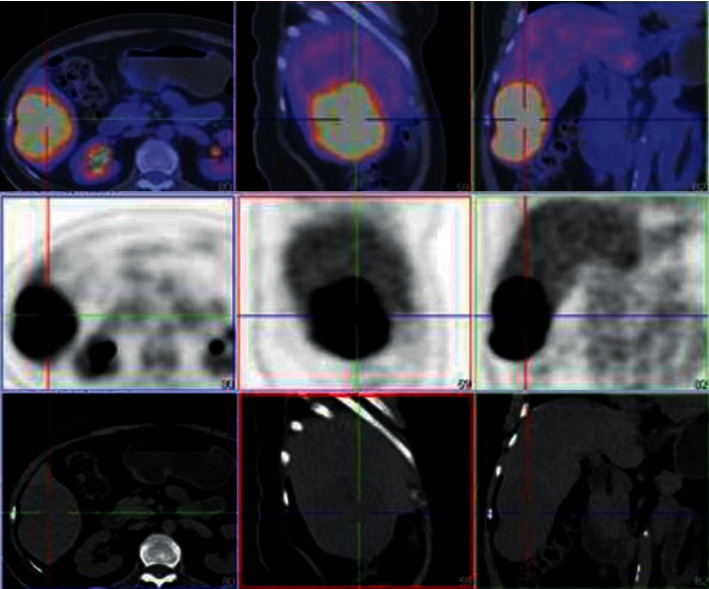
^18^FDG-PET-CT scan of Patient A on 6 July 2016. CT abdomen of Madam F on 9 August 2012. An 8 cm liver mass (SUVmax 8.4) was found at segment 8.
